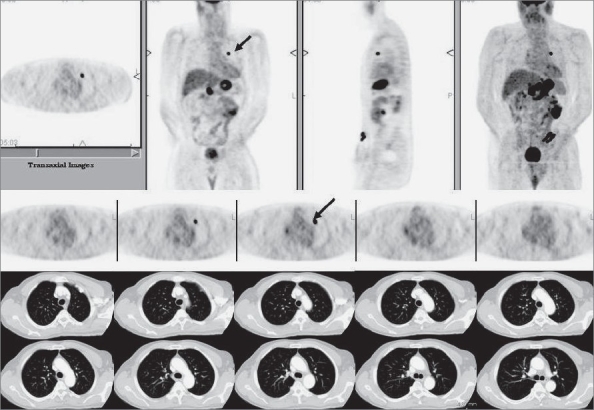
PET and CT scan images of a 64-year-old man with a descending/ sigmoid colon mass on recent colonoscopy. The PET scan reveals an intensely FDG-avid descending/ sigmoid colon malignant lesion with relatively widespread metastatic disease in the abdomen and a single intense left upper lobe metastatic lesion (arrows in the axial and MIP images in the top row and in axial images through the upper chest in the middle row). However, a contrast-enhanced chest CT scan done the next day reveals no corresponding lesion (bottom two rows), though there was evidence of pulmonary embolism
